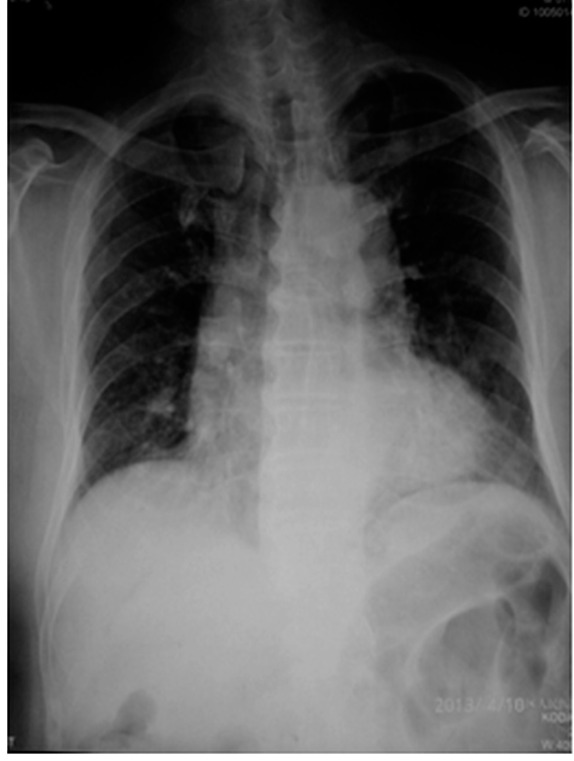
On April 3^rd^ 2013, the chest radiograph showed the left lower lung with a patchy, fuzzy shadow.
